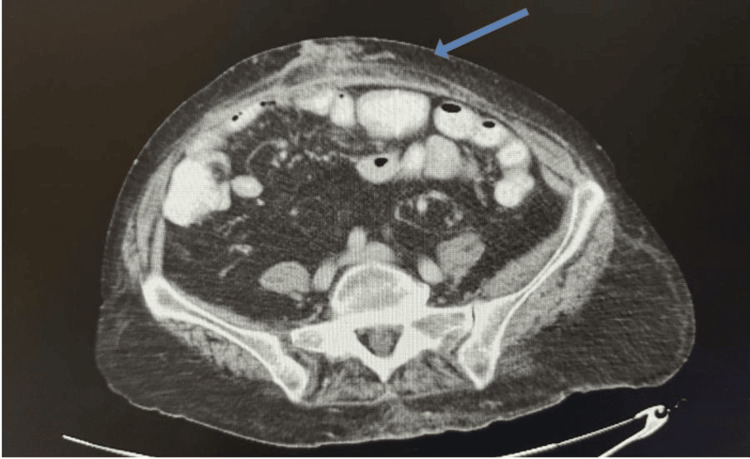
Postoperative CT scan following recto-rectus mesh repair and transverse abdominis release Blue arrow pointing to the repaired hernia
